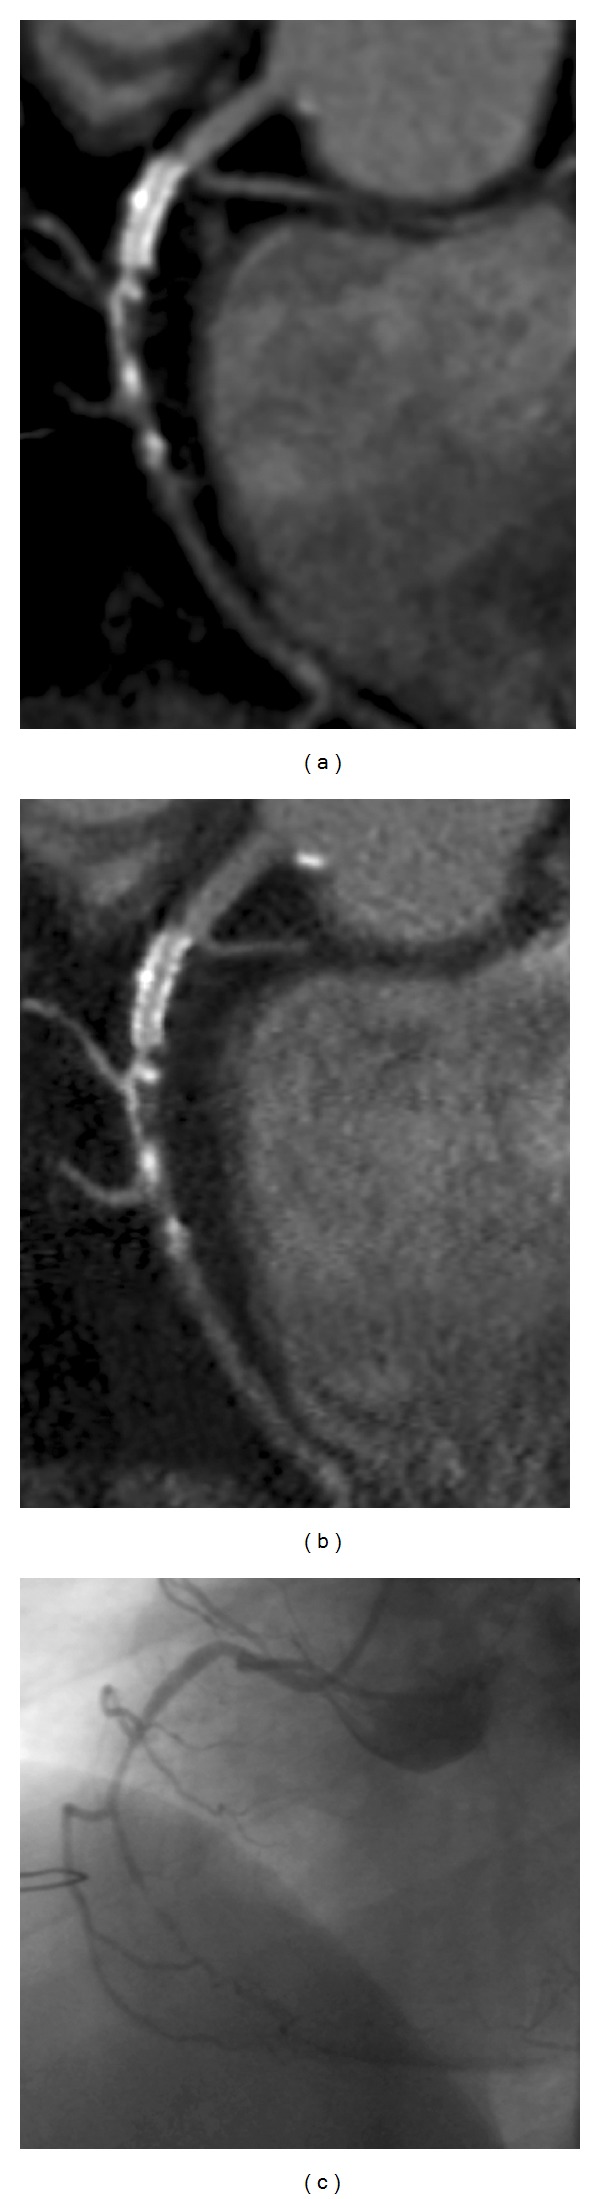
68-year-old male patient with a history of percutaneous coronary intervention with implantation of a 2.5 mm Xience V stent in the proximal RCA. CT shows the stent to be patent without relevant ISR, while there is a subtotal occlusion of the RCA distal to the stent (a, b). The finding was confirmed by coronary angiography (c). The stent lumen is better visible on images reconstructed with a dedicated convolution kernel (b), when compared with a standard convolution kernel (a). The use of a sharp convolution kernel goes along with a markedly increased image noise. Thus, the native vessel can be better assessed from images reconstructed with a smooth convolution kernel.
